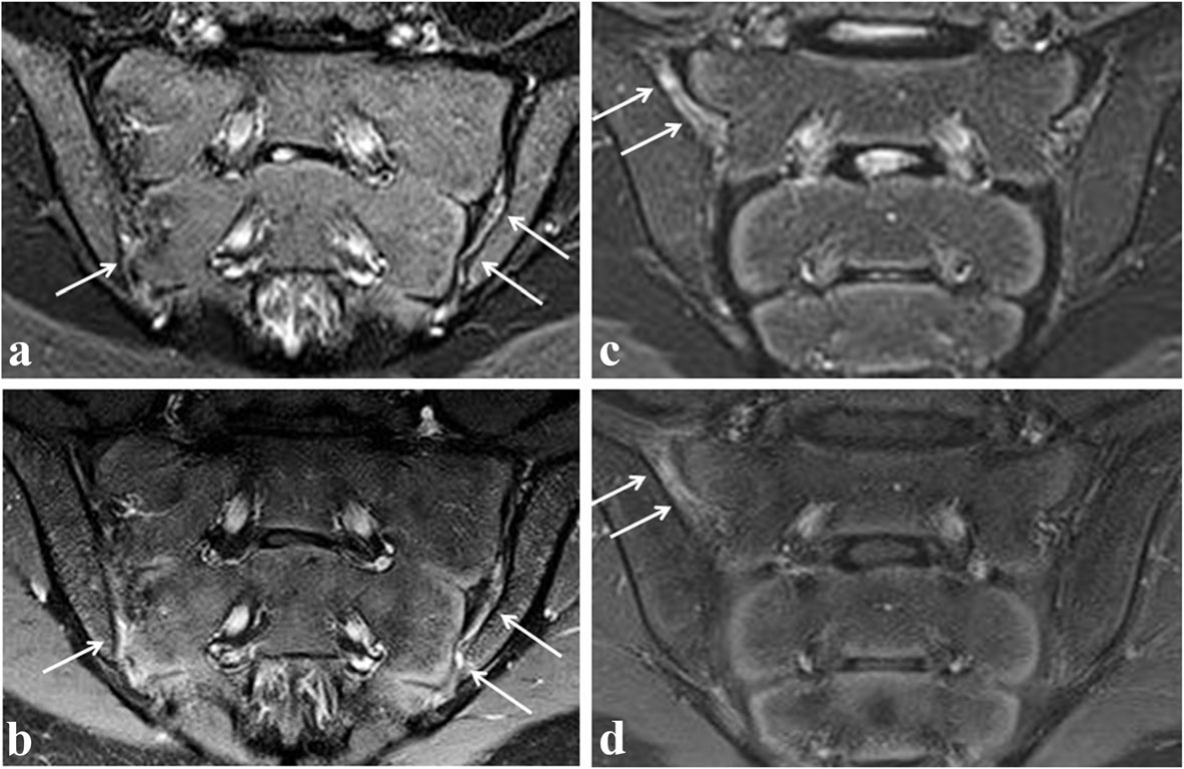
Active sacroiliitis in a 14-year-old girl (left) and a 14-year-old boy (right) with spondyloarthritis according to a global assessment of MRI for sacroiliitis, not according to the ASAS definition of a positive MRI for sacroiliitis. Semicoronal STIR (a and c) and contrast-enhanced fat-saturated T1-weighted (b and d) images on the left side showing synovitis in the caudal part of the left SI joint, also discrete in the caudal part of the right SI joint, seen as high signal in the joint space on STIR with corresponding synovial enhancement. Bone marrow edema is absent. On the right side, synovitis/retro-articular enthesitis is shown at the right sacroiliac joint (arrows). No BME is seen
